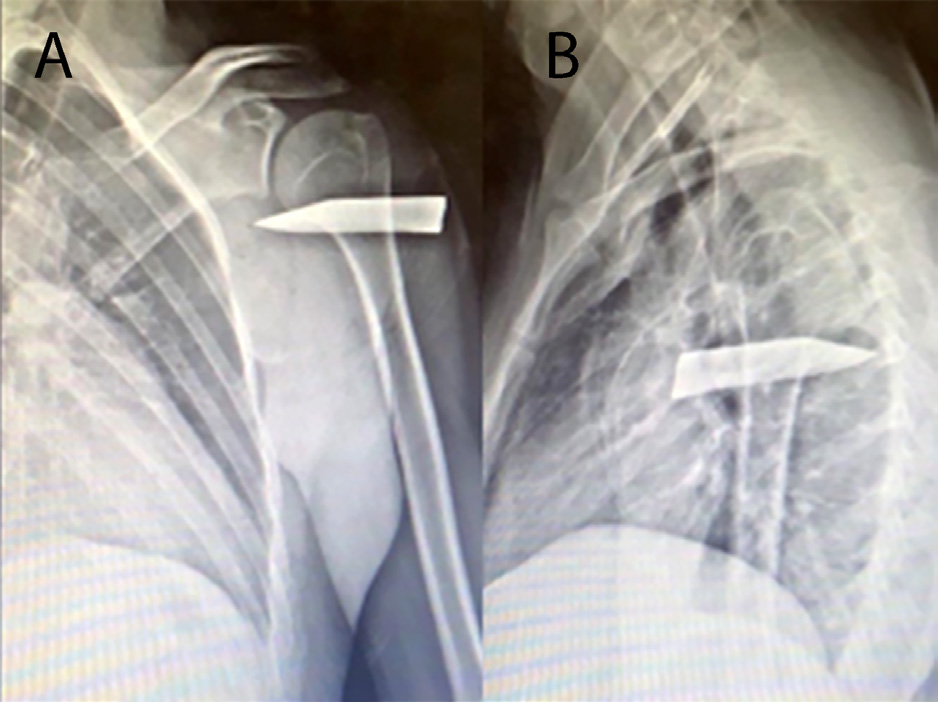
Anteroposterior and later radiography of the humerus demonstrating a foreign body penetrating the proximal humerus
